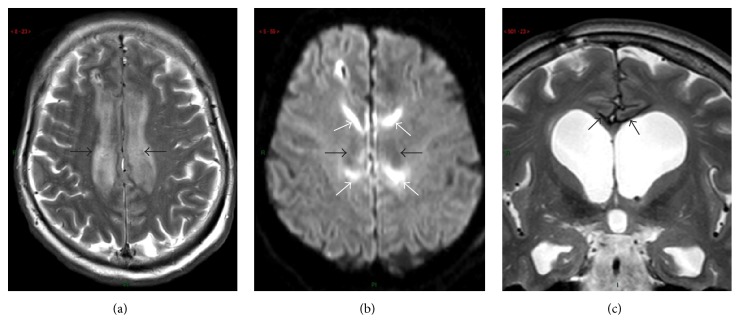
MRI work-up (a-b) at acute phase and delayed (7 months) MRI follow-up examination (c) of patient 1. (a) Axial transverse T2-weighted fast-spin echo (FSE) view through cingulate gyri demonstrating similar findings as in previous patient: oedema-related strong hypersignal intensity within them on both sides (black arrows). (b) Axial transverse diffusion-weighted (*b* factor = 1000 s/mm^2^) view in similar slice location (black arrows) failed to reveal hypersignal intensity, thereby excluding ischaemic cytotoxic damage. Only false positive artifacts due to adjacent hematoma were seen (white arrows). (c) Coronal T2-weighted FSE MR view at chronic phase (7 months) showed intrinsic textural integrity of the cingulate gyri. Meningeal hemosiderosis surrounding cingulate gyri featured by pial strong hyposignal intensity was present (arrows).
